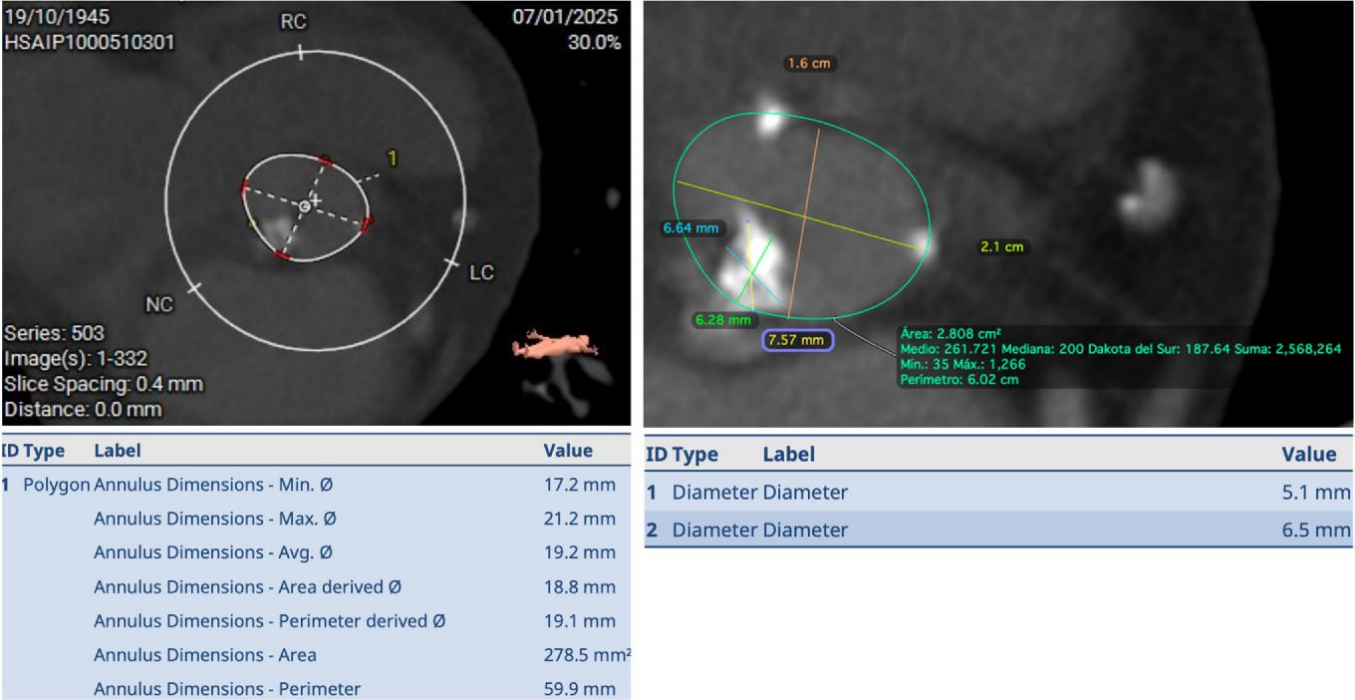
Preprocedural CT scan showing the annular measurement and calcium nodule.
